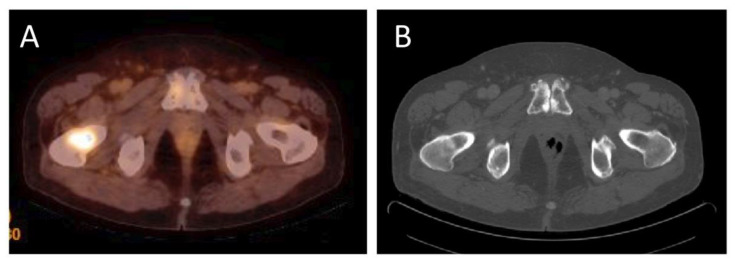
(A)—^18^F-PSMA-1007 PET/CT examination of a patient (69 years old) after radical prostatectomy seven years before, current PSA level 3.9 ng/mL. ^18^F-PSMA-1007 uptake in the right femur with SUVmax = 5.7 was detected to approve metastatic activity. (B) in computed tomography mild, local sclerotic lesion in the right femoral neck was inconclusive (Images from Riga Stradins University Radiology research laboratory archive).
